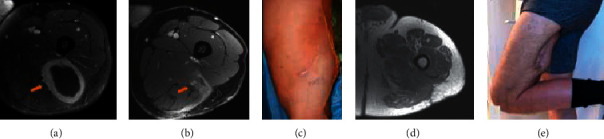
Axial MRI with contrast (a) showing a soft tissue sarcoma, involving the posterior compartment of the thigh abutting the sciatic nerve (arrow). The patient underwent a nononcologic resection at an outside center, and following the excision axial MRI with contrast (b) shows residual tumor abutting the sciatic nerve (arrow) and soft tissue edema involving the posterior compartment. In addition, they had an incision and surgical drain placed which did not follow oncologic principles (c). The resection included the sciatic nerve and the lateral hamstring complex and semimembranosus (d) with reconstruction utilizing a free functional latissimus with a skin paddle. Following surgery, the patient had full knee extension with flexion past 90° (e) ambulating without gait aid.
